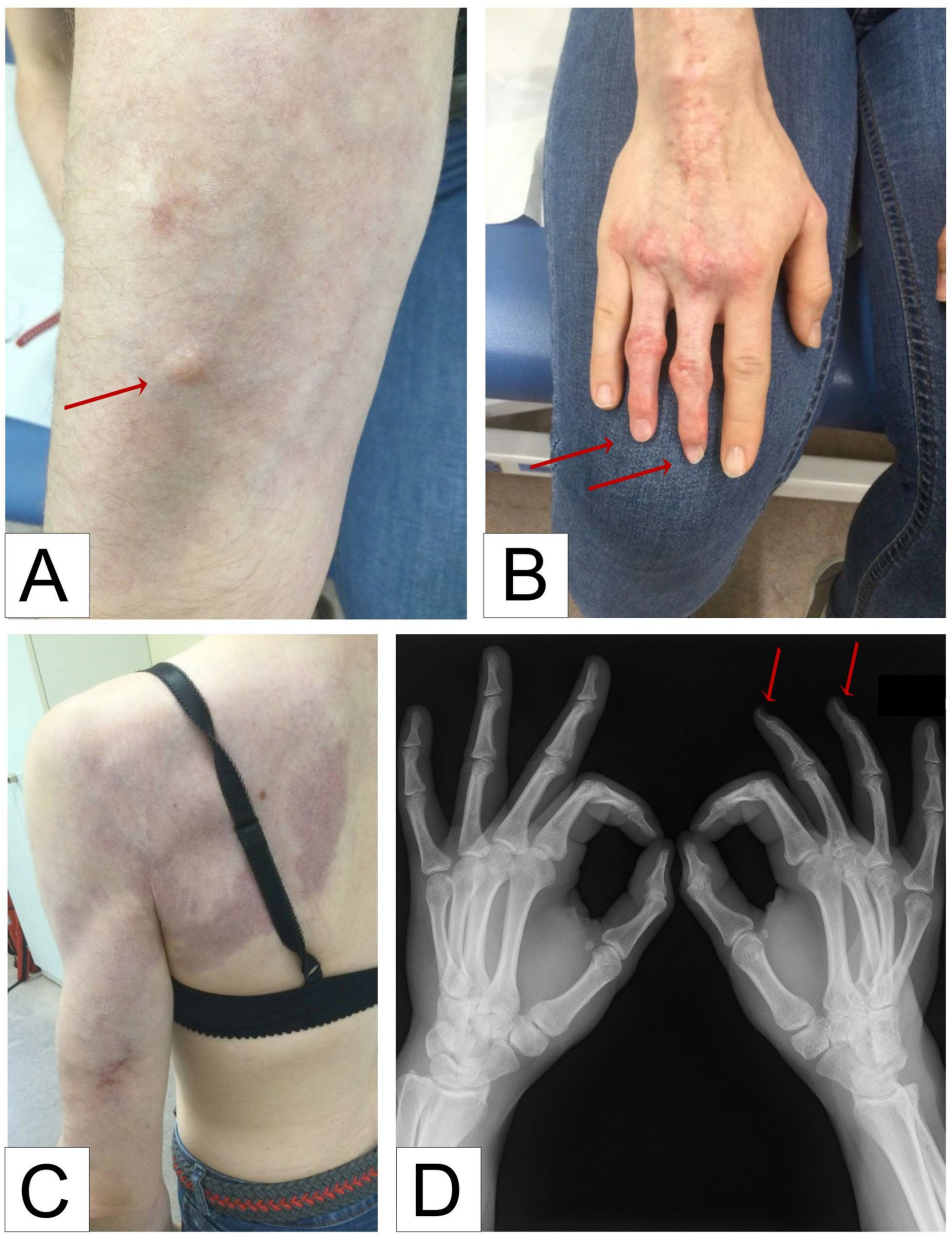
25-year-old young woman with multiple myofibromas and vascular anomalies, including (A) a superficial myofibroma of the right forearm, (B) congenital hypoplasia of the third and fourth finger of the right hand and (C) capillary malformation of the skin. (D) Xrays of both hands show distal mutilation of the metatarsal bones and the phalanges of the third and forth digit of the right hand
